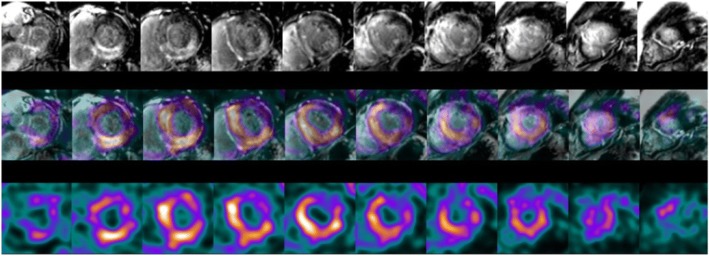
Positive Tc-HDP xSPECT images coregistered to delayed gadolinium enhanced short axis CMR images in a patient with ATTR cardiac amyloid showing the distribution within the myocardium. HDP uptake colocalises to sites of LGE (white regions within myocardium on CMR)
